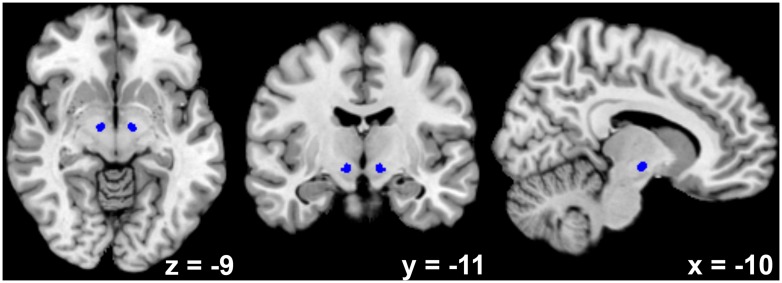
Depiction of the bilateral subthalamic nucleus seed region as derived from high-resolution susceptibility mapping using 7-T magnetic resonance imaging (Schafer et al., 2012).
